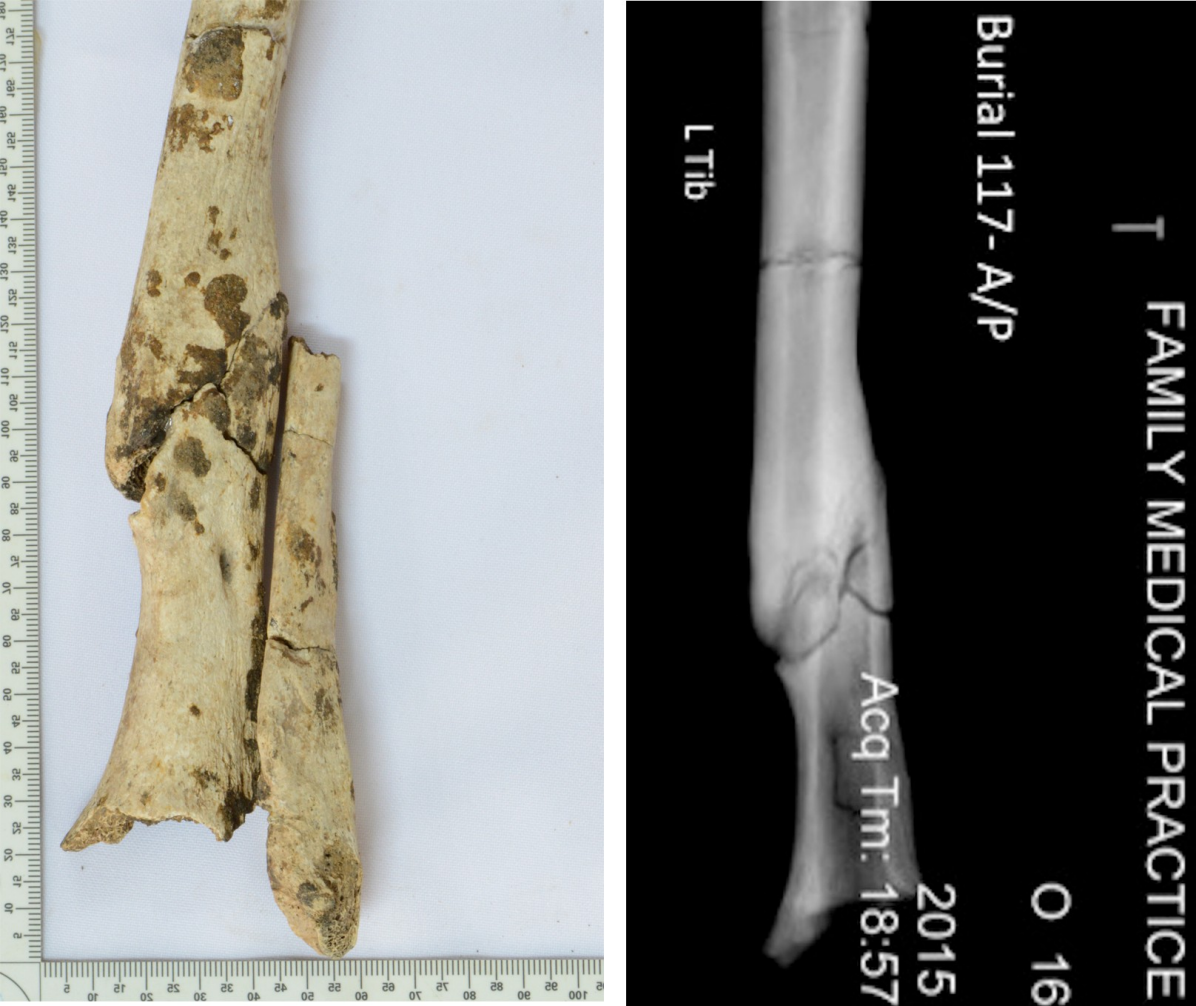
Left tibia and fibula (distal half of diaphysis, anterior view), and corresponding radiograph (tibia only, anterior view) of a young adult male buried at Con Co Ngua (Burial M117a). Note oblique fracture angle (in radiograph) and residual callus formation on tibial diaphysis].
